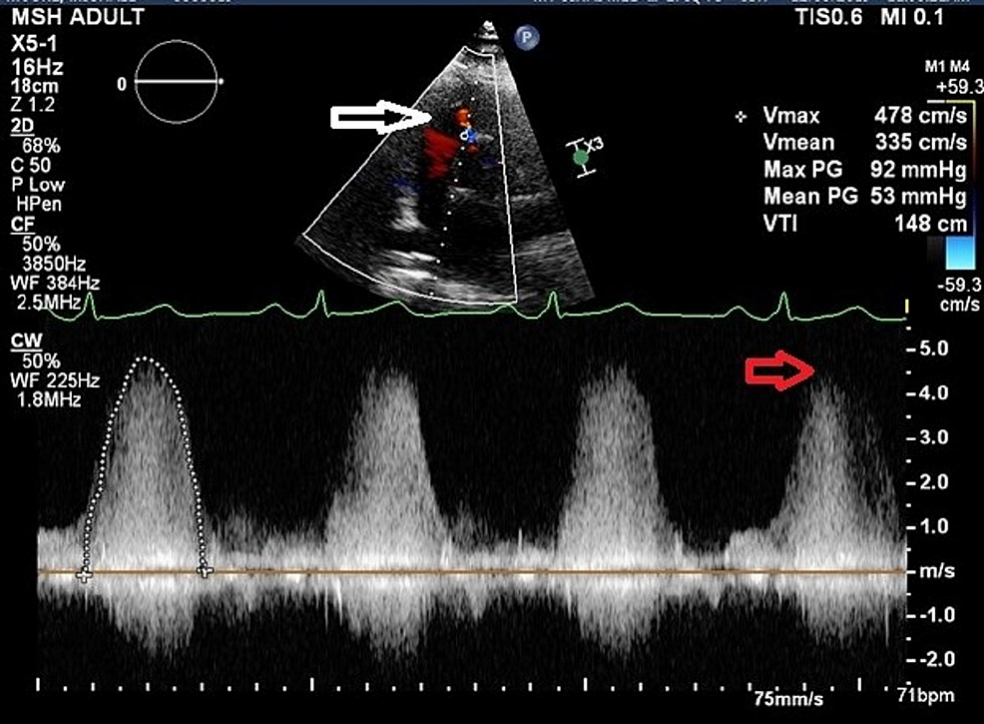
Transthoracic echocardiogram: VSD jet was identified to be around 4 m/sec, with the white arrow showing VSD and red arrow indicating velocity VSD: ventricular septal defect
